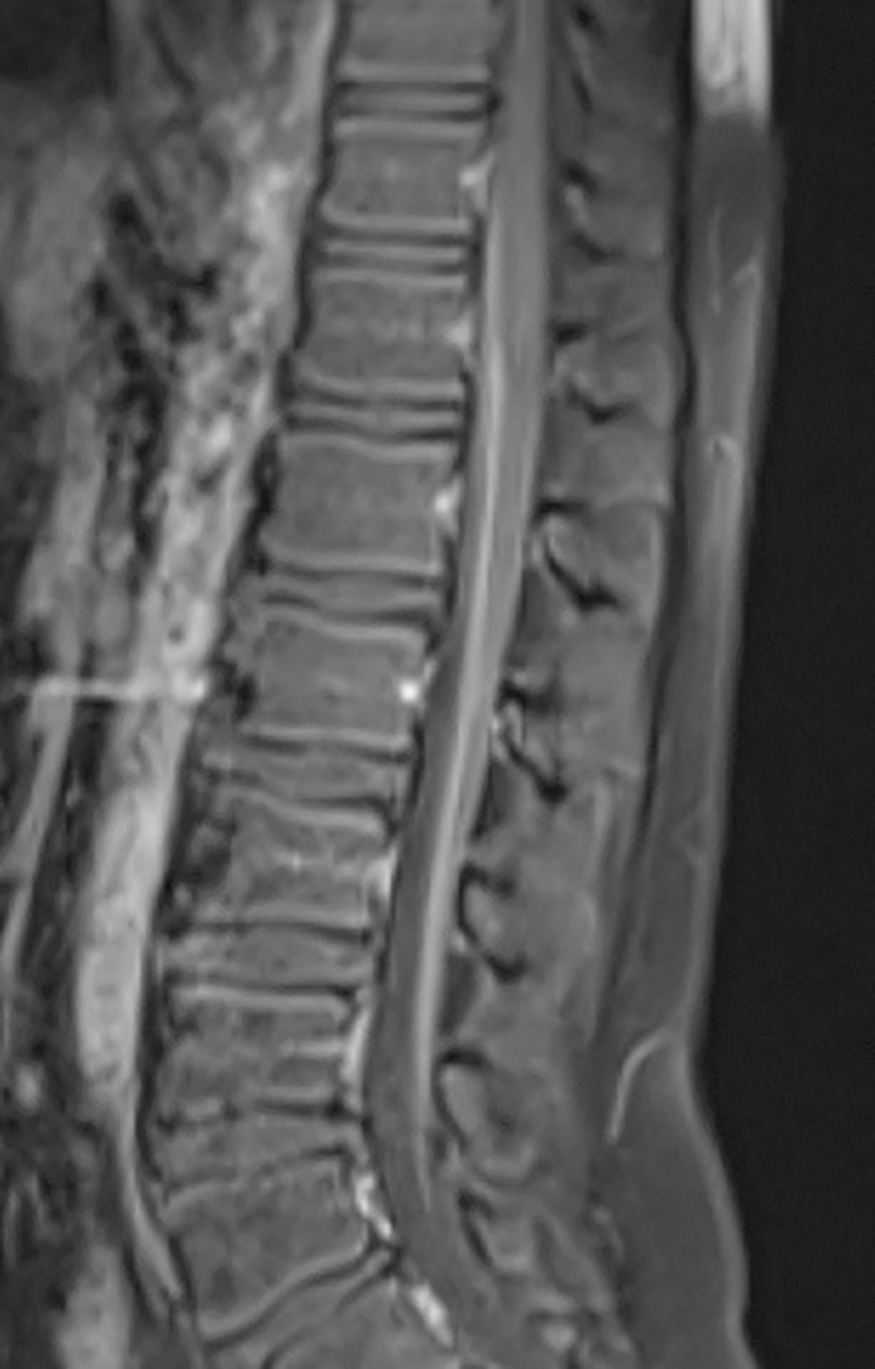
Sagittal T1-weighted post-contrast MRI of the spine demonstrates enhancement of the cauda equina nerve roots.
